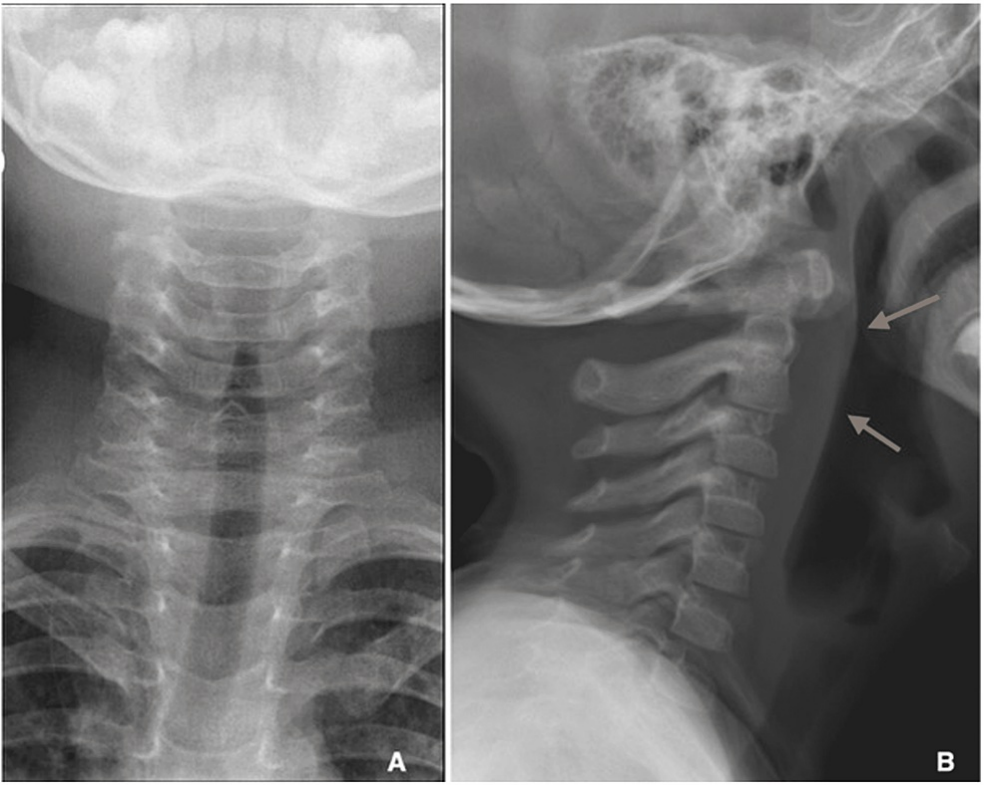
Coronal (A) and lateral (B) standard radiographs of the cervical spine showing non-specific thickening of the prevertebral soft tissues (arrows).
